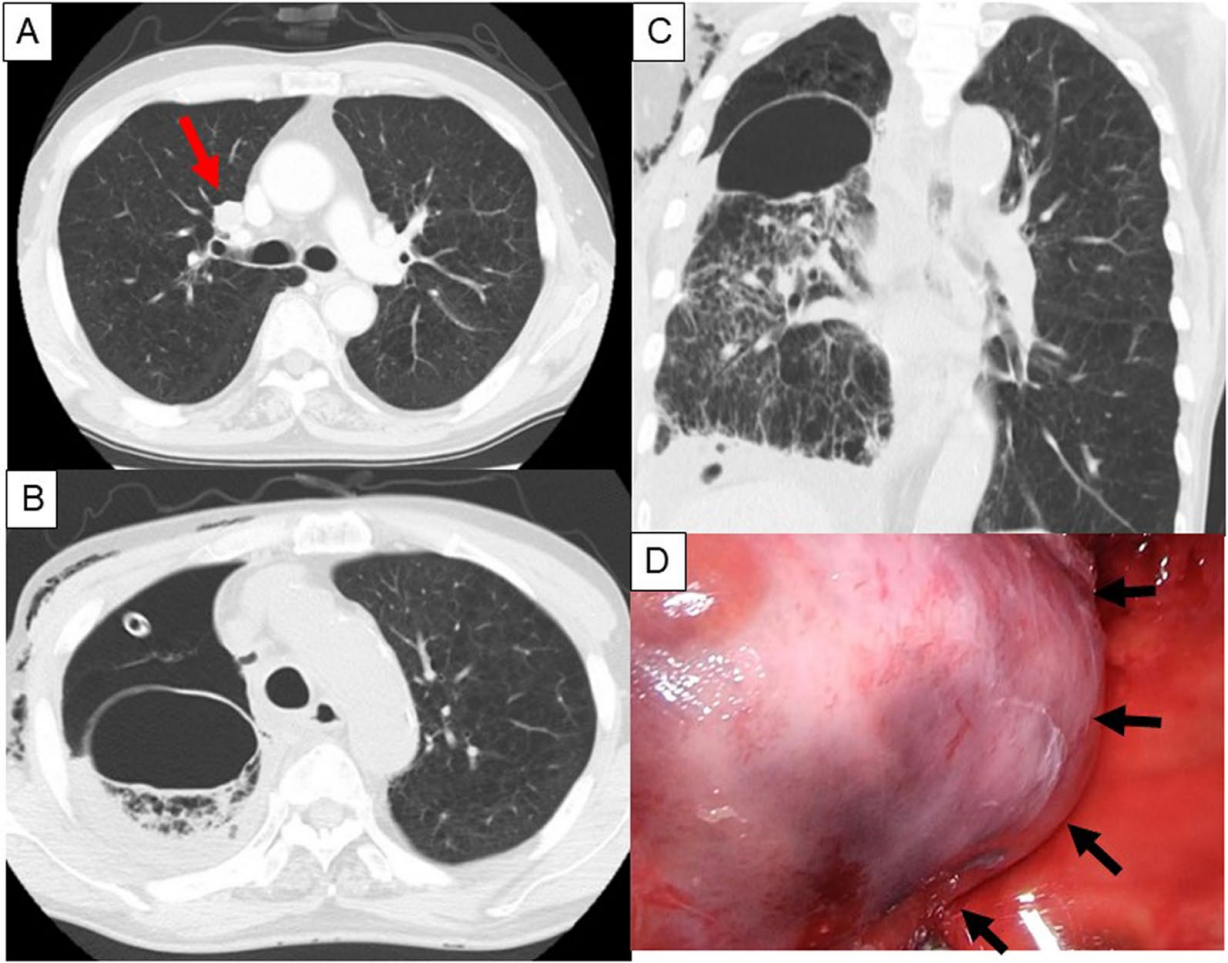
Preoperative CT and newly formed pulmonary cyst in Case 2. Preoperative CT shows a nodule measuring 16 mm in the right S3 (arrow) and severe emphysematous changes (A). Postoperative CT performed on POD 17 reveals newly developed pulmonary cyst at apex of S6 (axial section: B, coronal section: C). Broad-based pulmonary cyst with hole is recognized during reoperation (arrows) (D)
